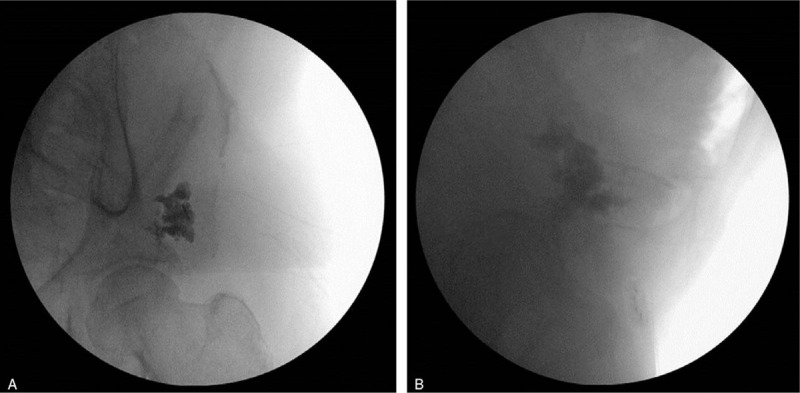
Left pelvic radiographs obtained immediately after the procedure using C-arm fluoroscopy in the operating room. Anteroposterior view (A) and oblique view (B) show the injected bone cement in left ileum.
